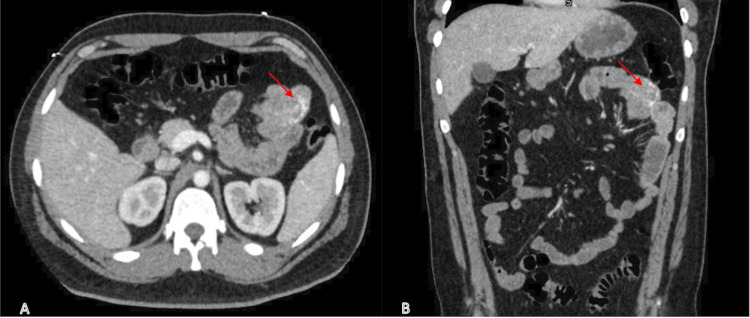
(A) Axial and (B) coronal contrast-enhanced CT enterography demonstrating an area of serpiginous enhancement in the jejunum (left upper quadrant) suggestive of active hemorrhage. CT, computed tomography
